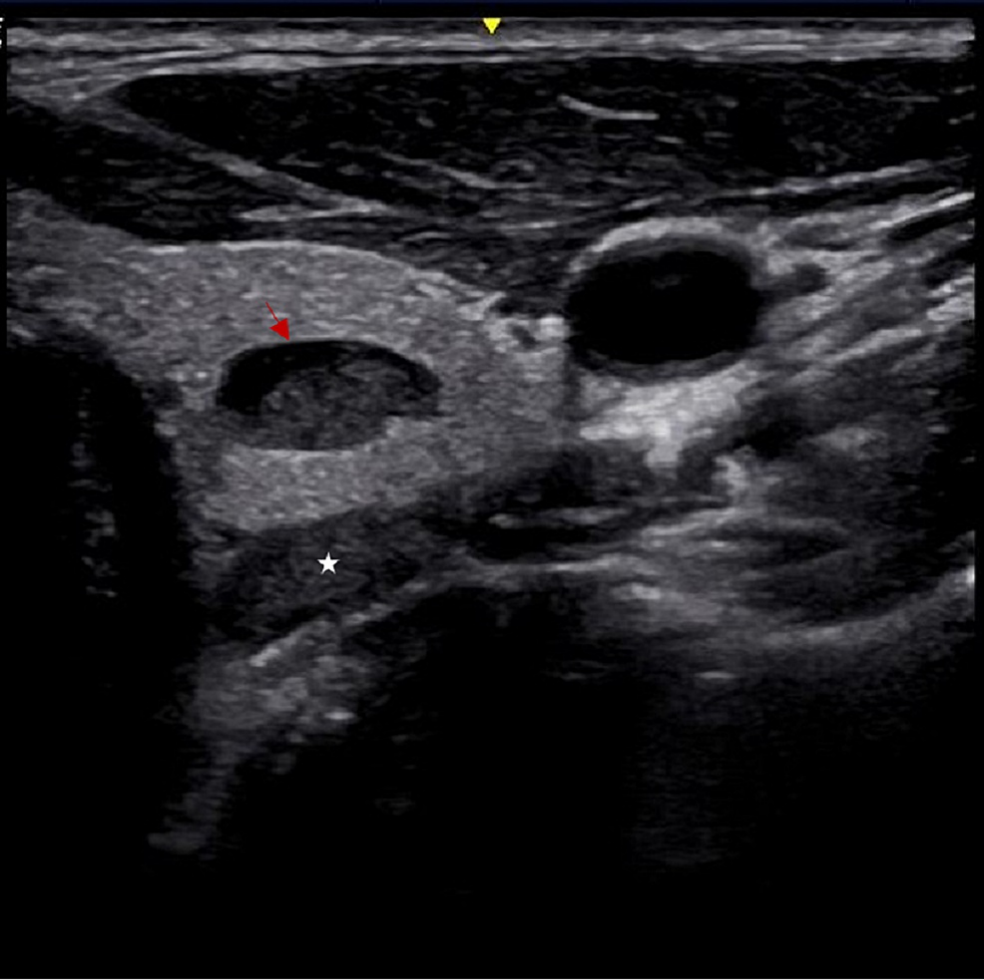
Ultrasound image of the left thyroid area during US-FNAB An ultrasound examination during US-FNAB revealed a hypoechoic cystic lesion (red arrow) in the left thyroid. The margins were smooth and well-defined. A few echogenic granules were observed in the capsule. An FNAB was then performed with two needle passes of a 21-gauge needle. A hypoechoic solid lesion (white star) corresponding to the site of the left parathyroid was also identified but not biopsied. US-FNAB - ultrasound-guided fine-needle aspiration biopsy
